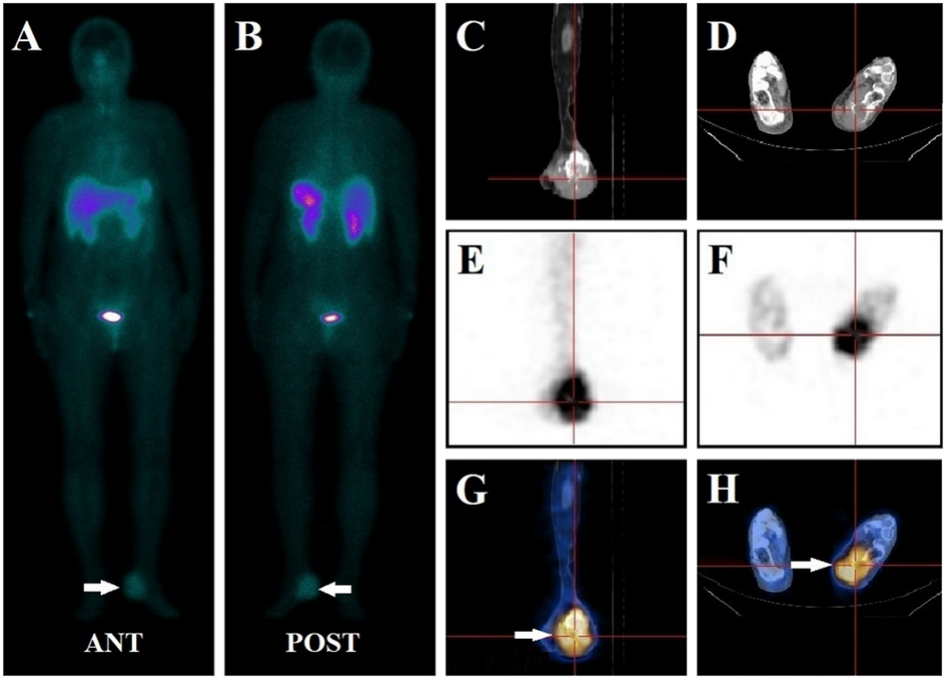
A 67-year-old female had left foot pain for more than two years. Her serum phosphate level was 0.79 mmol/L at presentation. The whole-body ^99m^Tc-HYNIC-TOC scan (A, B) demonstrated abnormal activity foci in the left foot (white arrow). SPECT/CT images revealed focal intense uptake in the left calcaneus bone in SPECT (E, F) and fused SPECT-CT images (white arrow) (G, H). CT scans (C, D) identified bone destruction with associated soft tissue swelling of the left calcaneus. The lesion was completely excised and confirmed to be a well-differentiated G2 neuroendocrine tumor. Pain symptoms ceased, and the patient’s serum phosphate levels increased to 1.17 mmol/L after oral phosphate supplementation. Thus, ^99m^Tc-HYNIC-TOC SPECT/CT detected foci were considered a false positive finding.
